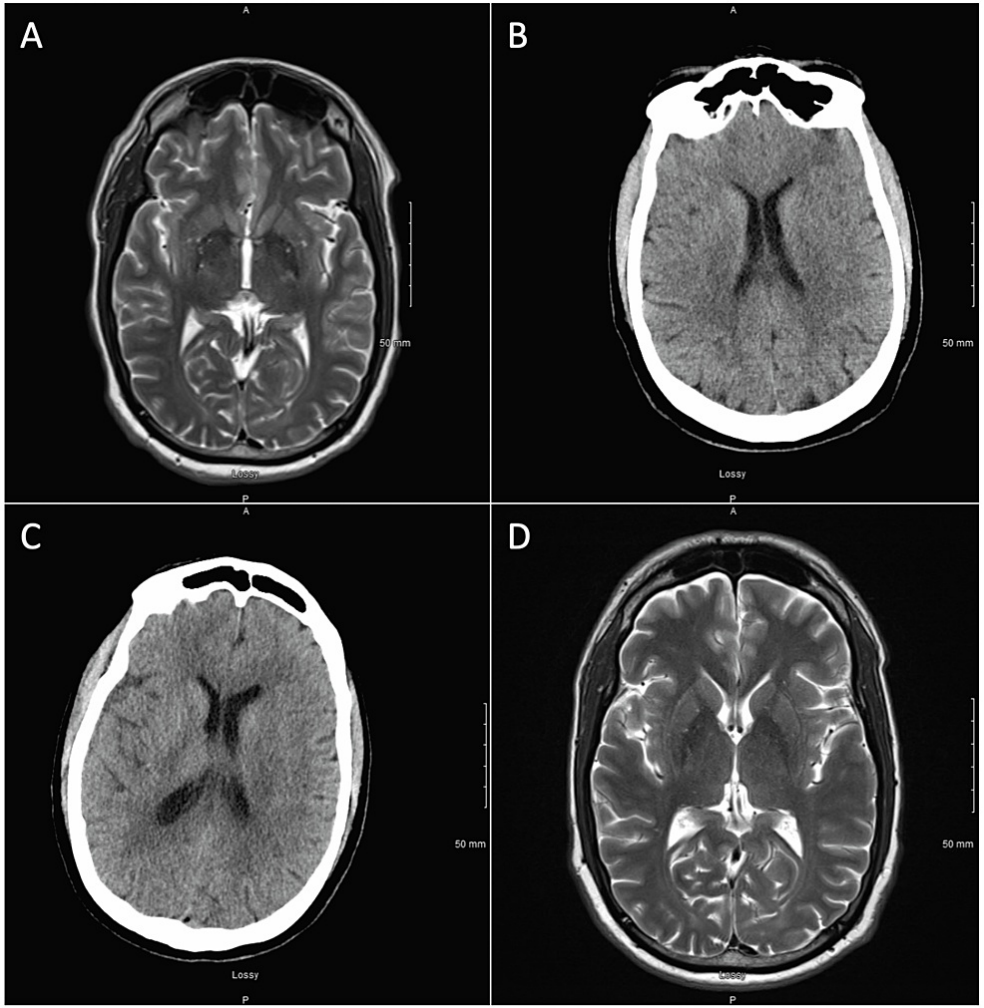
Imaging series by admission. (A) Unremarkable MRI brain on the first admission. (B) Unremarkable CT head without contrast on the second admission. (C) Unremarkable CT head without contrast on the third admission. (D) Unremarkable MRI brain on the fourth admission.
